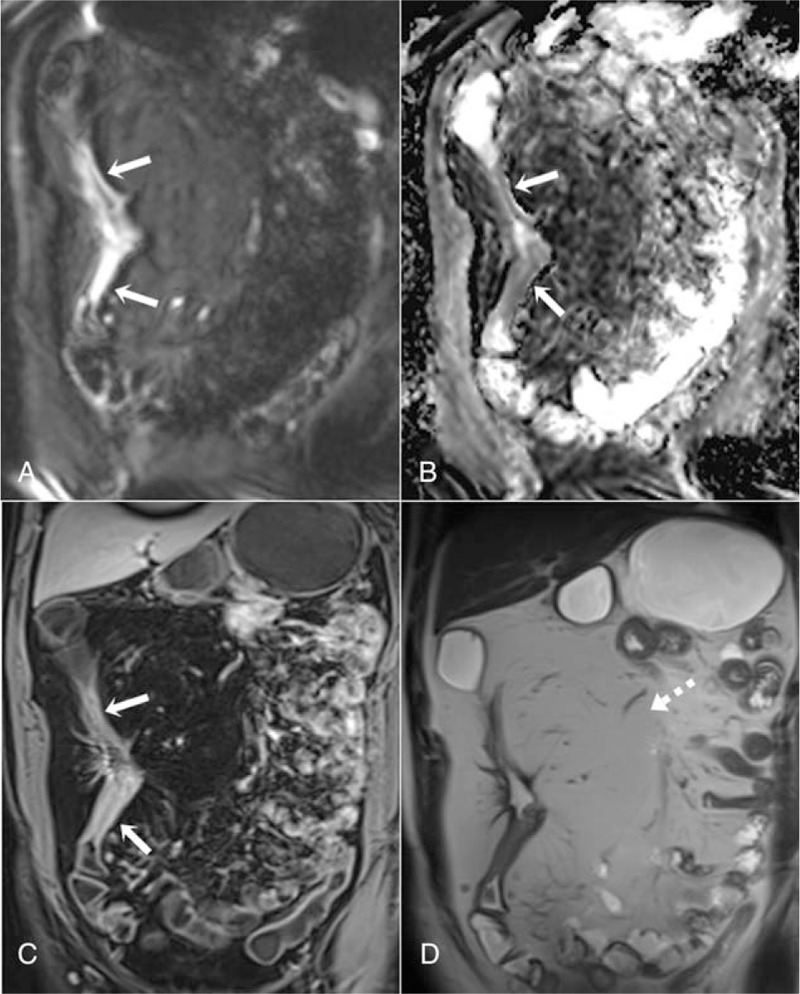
A 30-yr-old male patient with active Crohn disease on a chronic basis in the descending colon. Diffusion restriction is observed in the colon wall on coronal DWI (A) and the ADC map (B) (arrows). Wall thickness, increased contrast enhancement, and strictures are observed on the coronal contrast-enhanced T1-weighted sequence (C) (arrows). Fibrofatty proliferation exists neighbouring the pathological intestinal segment on the coronal T2-weighted images (D) (dashed arrow). ADC = apparent diffusion coefficient, DWI = diffusion weighted imaging.
